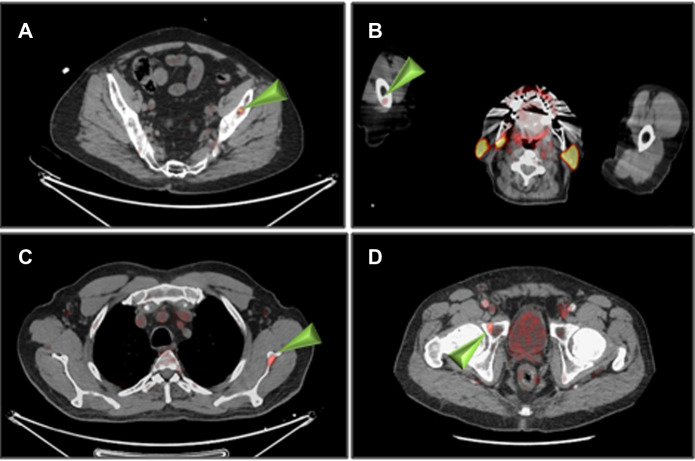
Non-rib skeletal uptake of [^18^F]PSMA-1007 (arrowheads) 1 h after injection. (A) patient #1 (left iliac), (B) patient #1 (right humerus), (C) patient #3 (left scapula), (D) patient #10 (right pubic bone).
